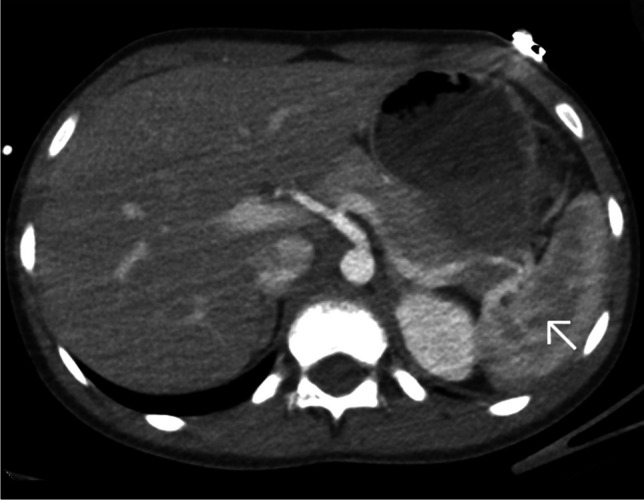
A 10-year-old girl with a history of a ski fall. Axial computed tomography, post-contrast, following a split-bolus protocol upper-abdominal image (soft tissue window). A too early acquisition resulted in suboptimal image quality with an inhomogeneous splenic enhancement (*arrow*), with alternating hypodense stripes mimicking splenic lacerations
